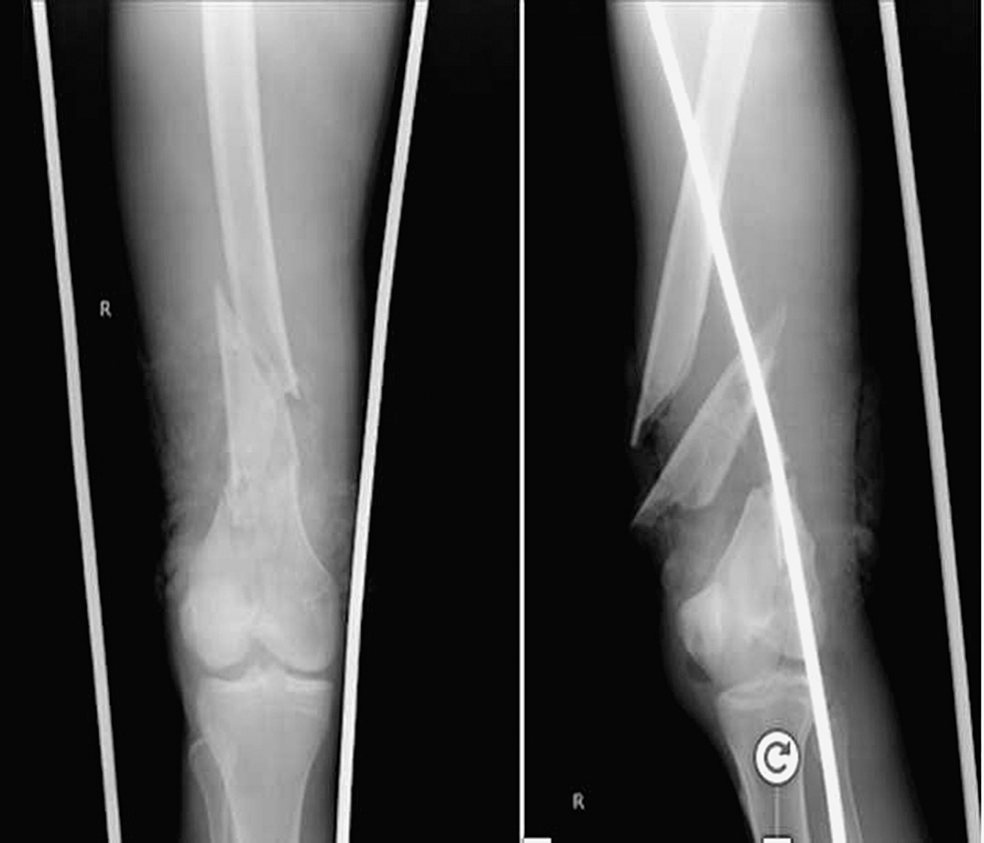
X-ray right femur showing segmental supracondylar femur fracture
